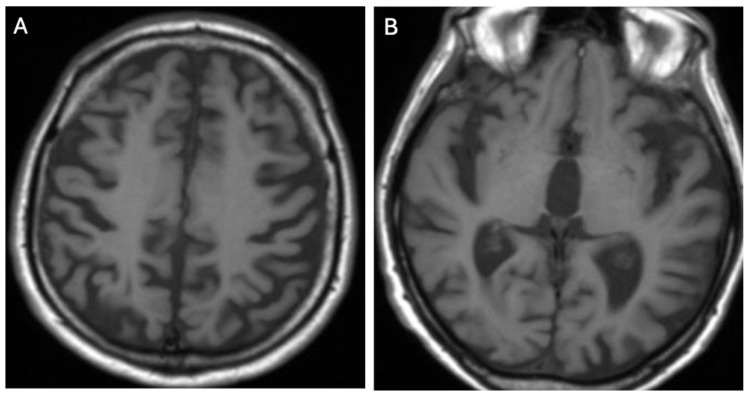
Cortical atrophy (A) and central atrophy with widening of the third ventricle (B) in T1-weighted sequences (own materials of the neurology department).
